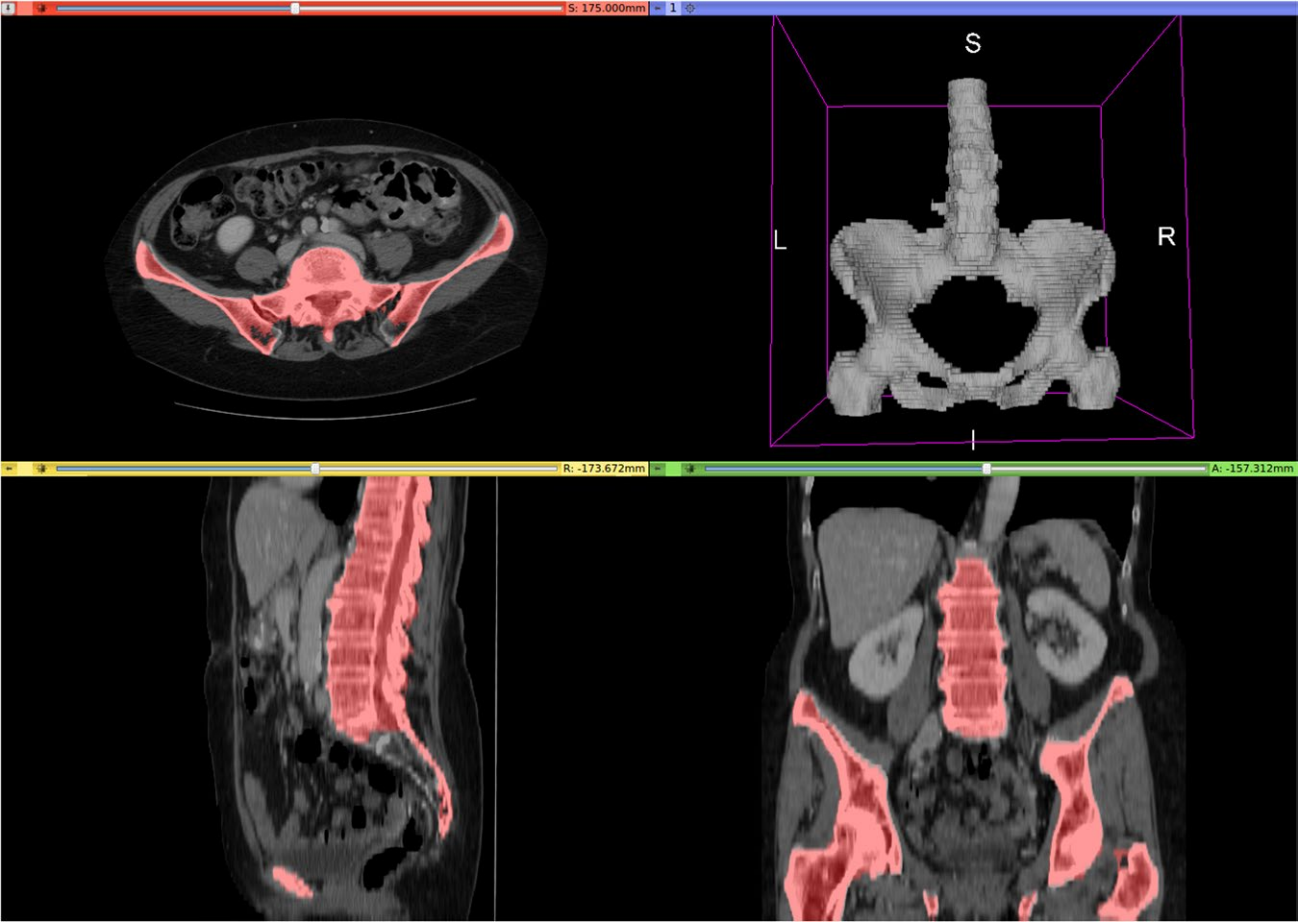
Example of CT skeleton detection and segmentation by image morphology. Skeleton mask overlaid in red. Rendered by 3D Slicer^24^.
